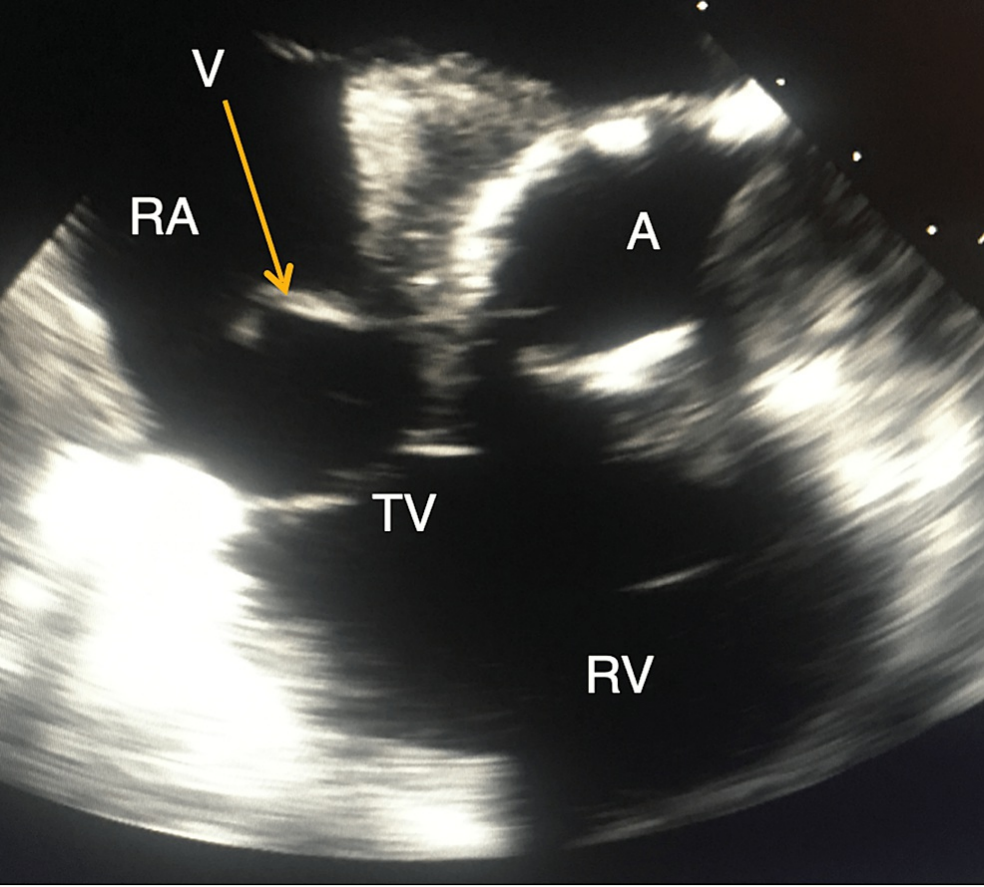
Transesophageal echocardiogram (TEE) TEE showing vegetation. (A = Aorta, RA = Right Atrium, RV = Right Ventricle, TV = Tricuspid Valve, V = Vegetation)
